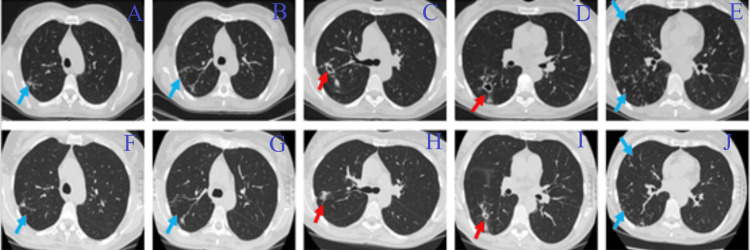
CT scan of patient 2 Figure panels A, B, C, D, and E present imaging before treatment, and panels F, G, H, I, and J present imaging at the end of the initial phase of treatment. A decrease in the size of the cavities (red arrows) and a decrease in the extent of tree-in-bud lesions (blue arrows) are observed.
